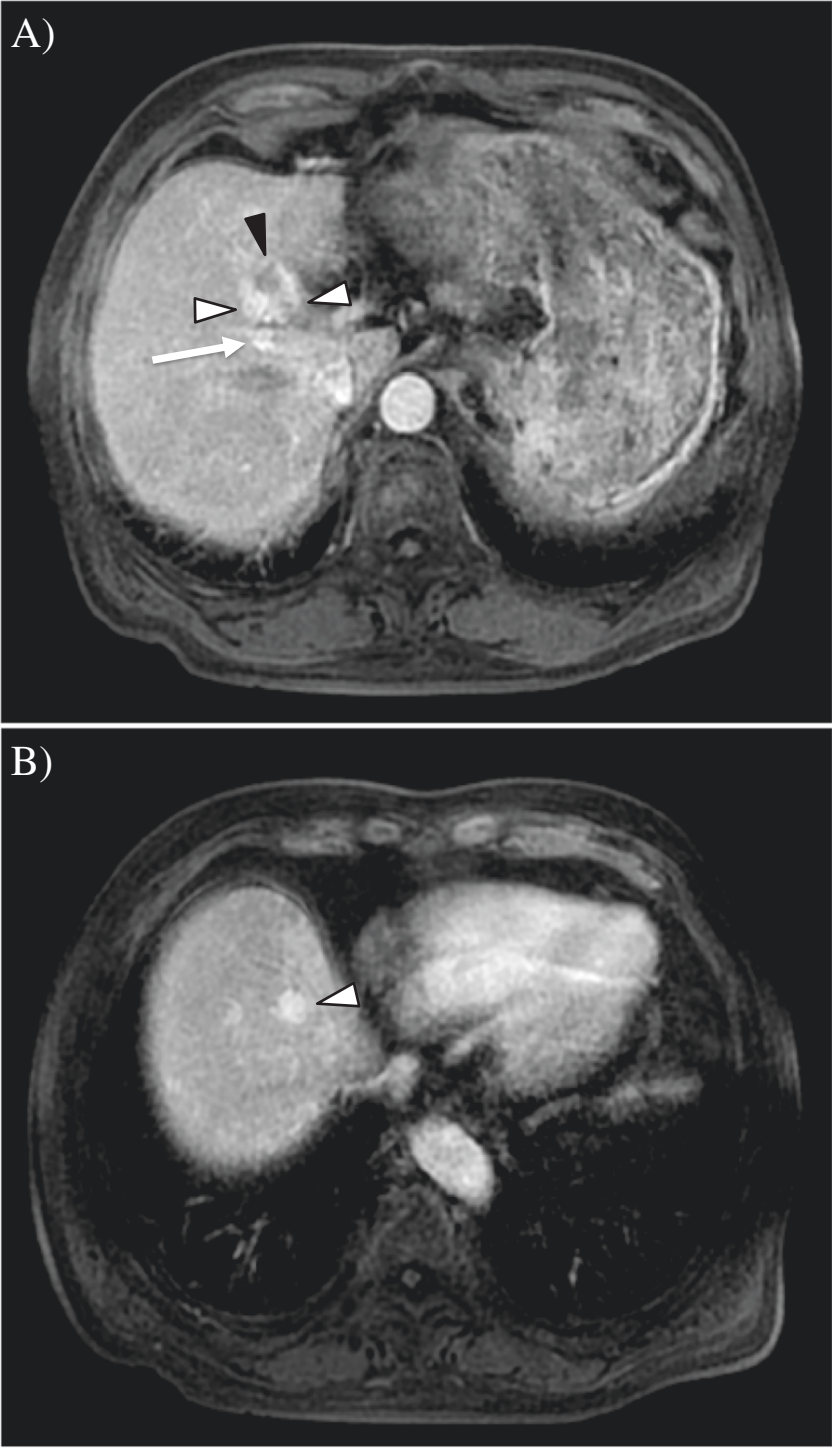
Gadoxetic acid-enhanced magnetic resonance imaging at tumor discovery. Images in the arterial-phase are shown. (A) A large tumor, 23 mm in diameter, is adjacent to the right Glisson branch (white arrow). The dorsal side of the tumor is hypervascular (early enhancement is indicated by white triangles), and the ventral side is hypovascular (indicated by a black triangle). (B) A smaller tumor, 13 mm in diameter, is below the right diaphragm. The tumor also shows early enhancement (indicated by a white triangle).
